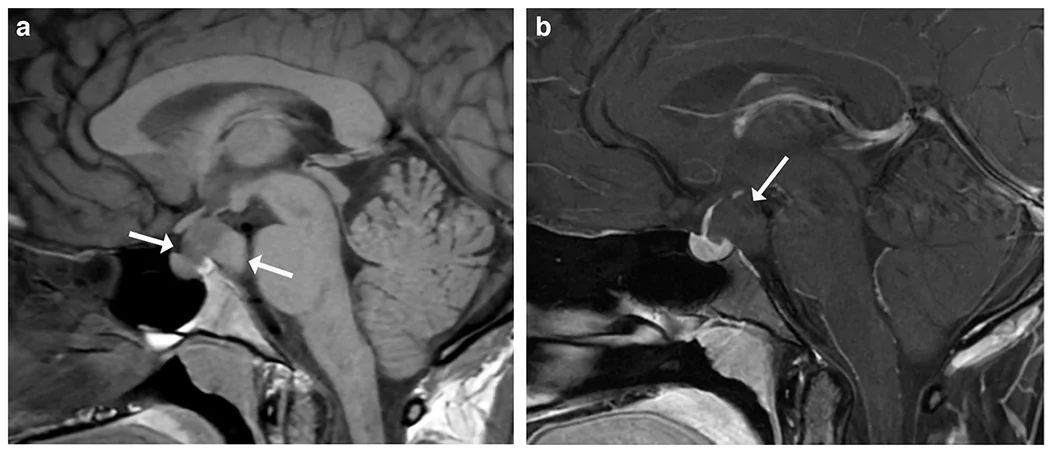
Rathke cleft cyst in an 18-year-old female with diplopia and elevated prolactin levels. Sagittal T1-weighted images acquired (a) pre-contrast and (b) post-contrast show a large, exophytic cystic lesion with a fluid-fluid level (*arrows* in a) hanging over the dorsum sella. Post-contrast image accentuates the infundibulum, displaced anteriorly by the mass effect of the cyst (*arrow* in b)
